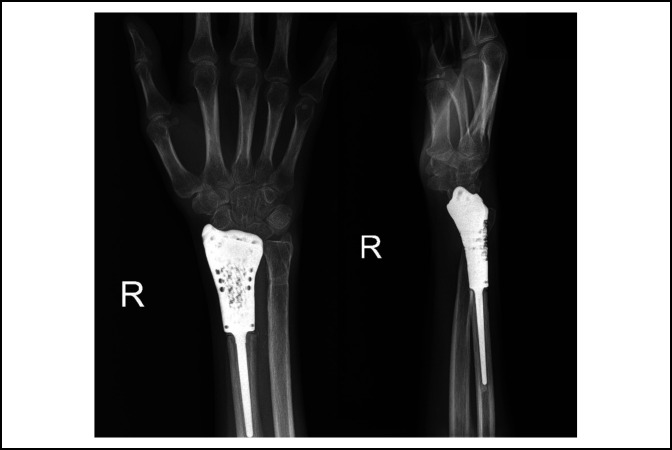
Postoperative x-ray of patient after 18-month follow-up in PA and lateral views.
